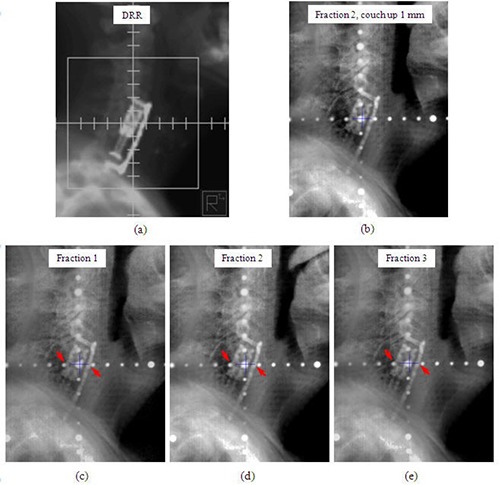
Image‐guided setup of a spinal SRT patient (3 fractions). The right‐lateral DRR reference image (a) was compared with the MV portal images (c), (d), and (e) acquired immediately following CBCT‐based patient alignment. There was an estimated 1 mm offset (red arrows) in the AP direction between DRR and MV portal images. A new MV portal image (b) was taken after applying a couch shift (raising couch 1 mm) in fraction 2. The blue crosses in the MV portal images were digital graticules superimposed by the record and verify software and were not relevant in this study.
